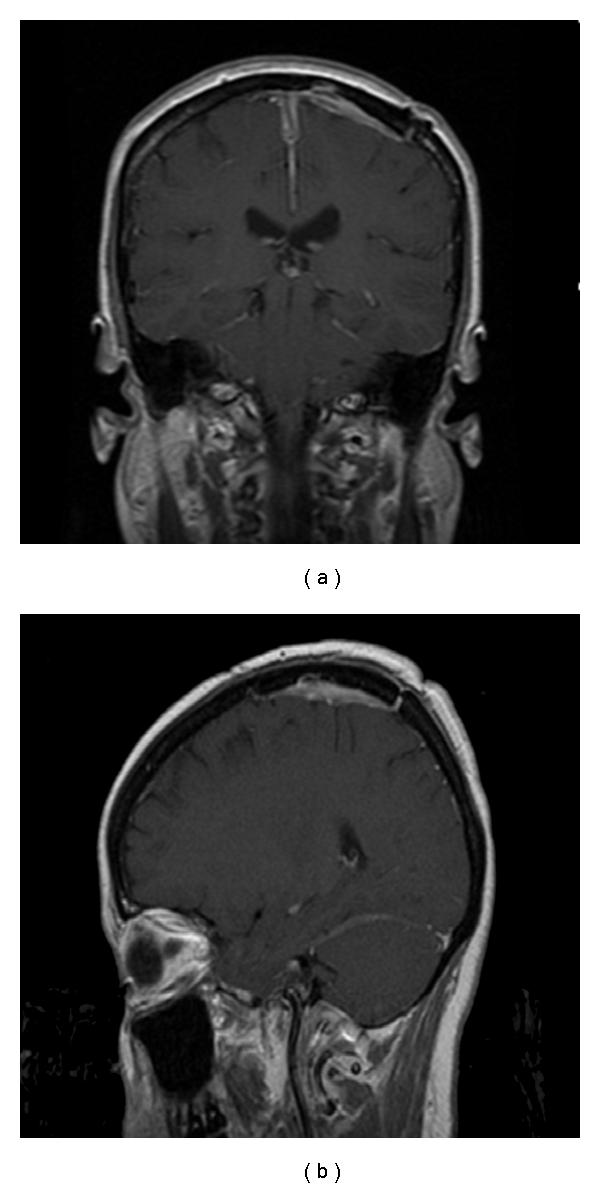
MRI posttreatment showing resolution of the disease with postsurgical changes.
